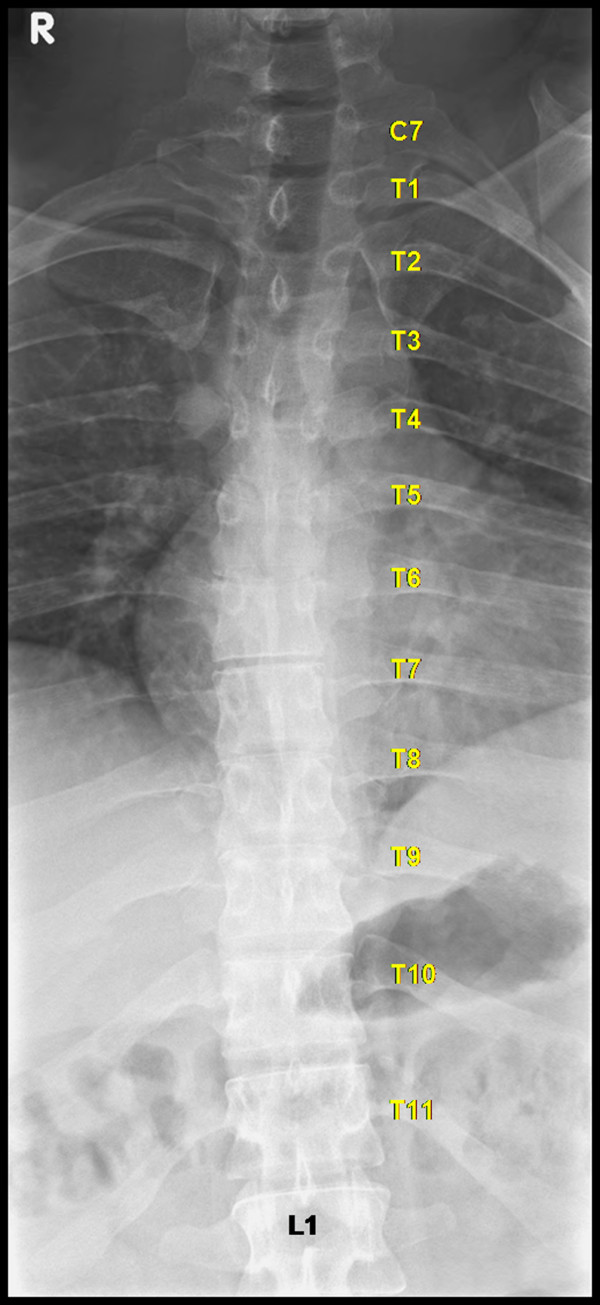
Thoracic rib counting. Antero-posterior radiographs of the thoracic spine demonstrate eleven pairs of true thoracic ribs.
